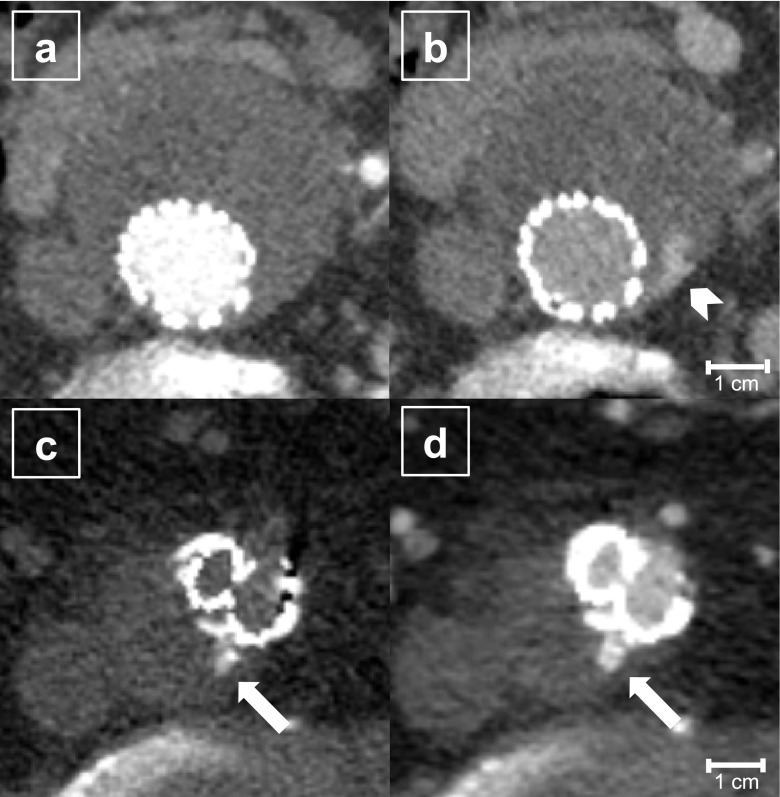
An 82-year-old EVAR patient (m) undergoing follow-up with a triphasic CT scan (level 100 HU, window 600 HU) 1 year after intervention. The arterial phase (a) and venous phase (b) show a low flow endoleak (arrowhead) apparent only in the venous phase (b). The native scan (c) identifies hyperdense material within the aneurysm sac as calcifications (arrows). These could be mistaken as an endoleak in the contrast-enhanced scan (d)
